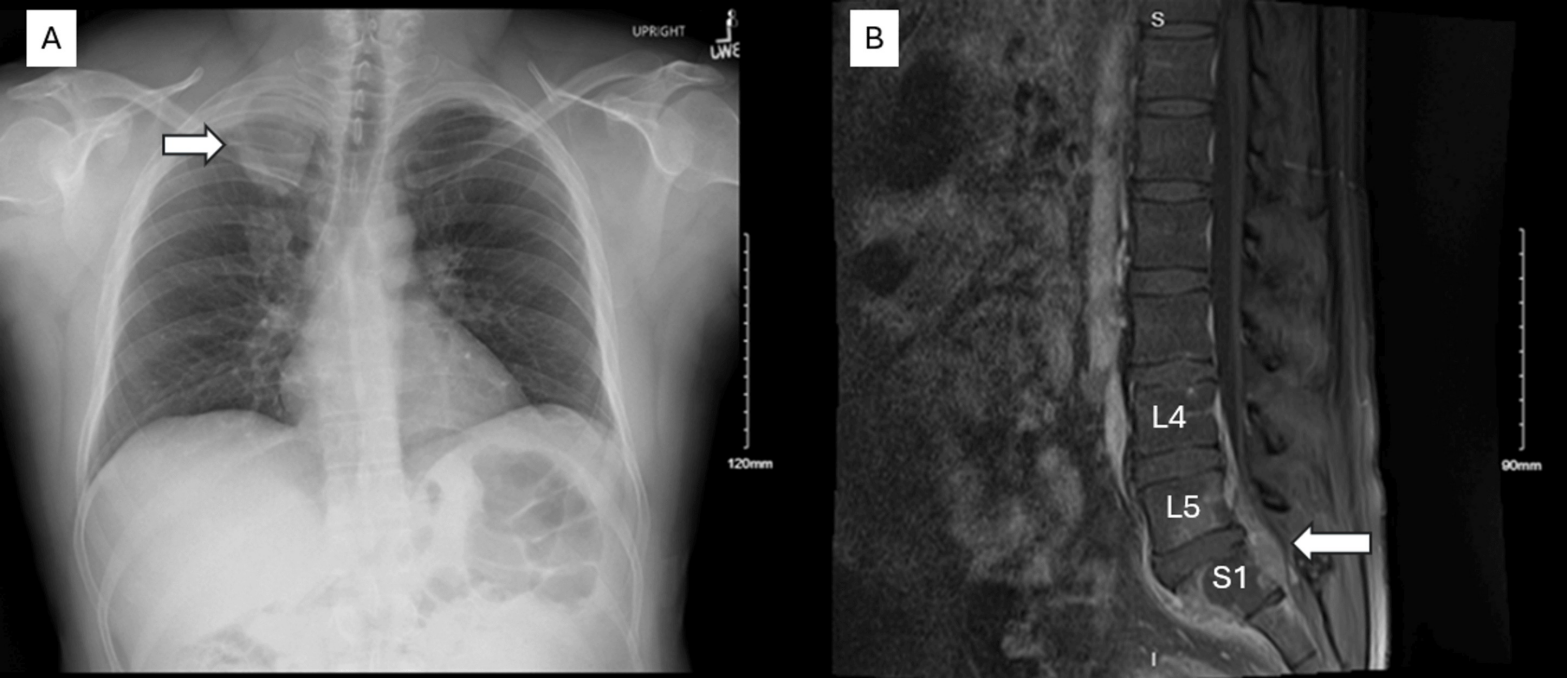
A) Upright chest X-ray on presentation, showing a right apical mass (arrow). B) Lumbar MRI with contrast (T1-weighted), showing significant stenosis at L5-S1 (arrow).
